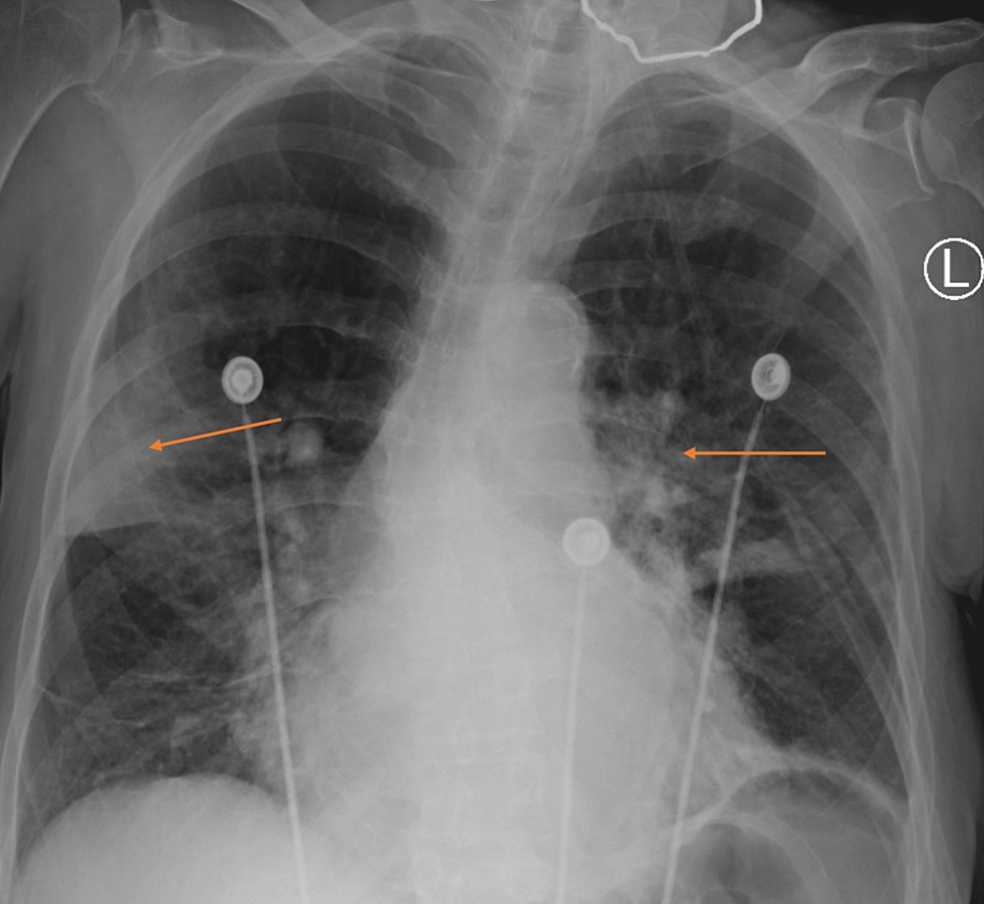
Repeat Chest X-Ray Demonstrating Improvement in Bilateral Lung Opacification (arrows).
